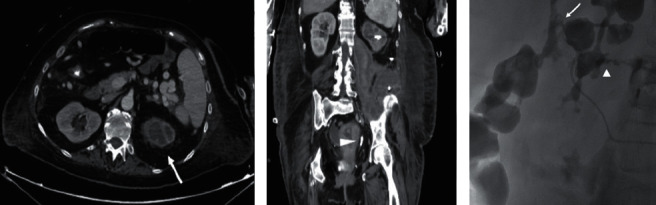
(a) Postcontrast CT images showed hypoperfusion of the left kidney with thinning of the cortex; dilatation of the left kidney excretory system with a “bear's paw sign” is also depicted suggesting diagnosis of xanthogranulomatous pyelonephritis (arrow); (b) postcontrast coronal reconstruction demonstrated ureteral stone (arrowhead) in the distal tract of the left ureter without significant dilatation of the proximal tract; (c) oblique projection pyelography after puncture of the inferior calix of the left kidney demonstrated leakage of contrast media with a double fistulous path form the pelvis directed anteriorly to the left colon (arrow) and posteriorly to the collection in the retroperitoneal collection (arrowhead).
